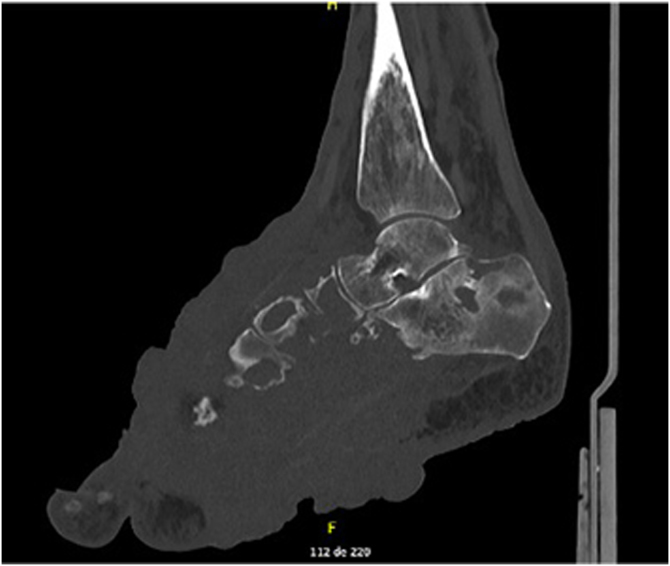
Computed tomography of the right foot showing voluminous expansive and infiltrative solid tissue with extensive bone and soft tissue involvement.
